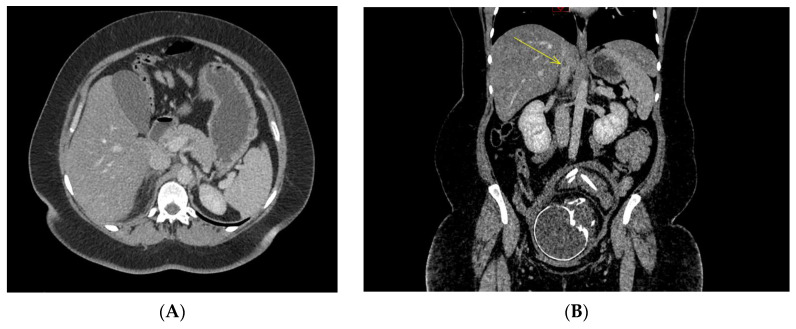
CT-scan sections ((A) infiltration of the right adrenal compartment with edematous appearance and overall hypo-enhancement of the right adrenal gland; (B) yellow arrow indicates venous thrombosis of the inferior vena cava complicated by hemorrhagic necrosis of the right adrenal gland).
